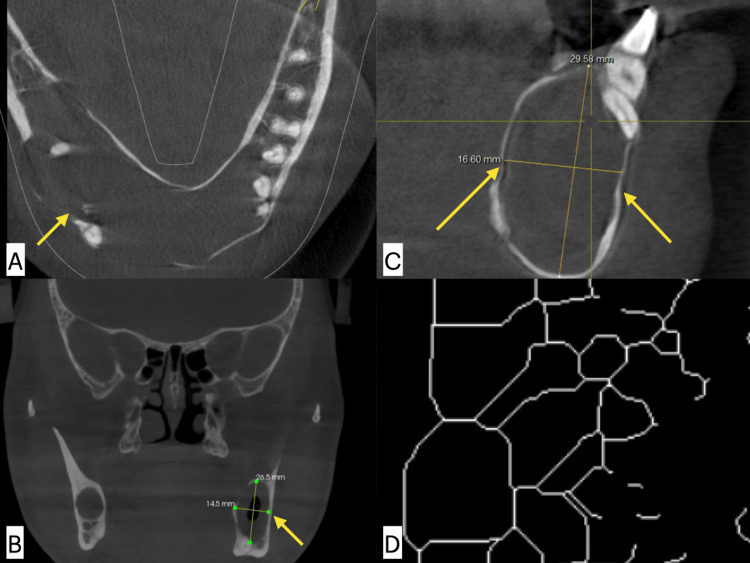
Odontogenic keratocyst (OKC) in CBCT images measurement of the size of the lesion in (A) axial, (B) coronal, (C) sagittal plane, and (D) final fractal image. CBCT: cone beam computed tomography
